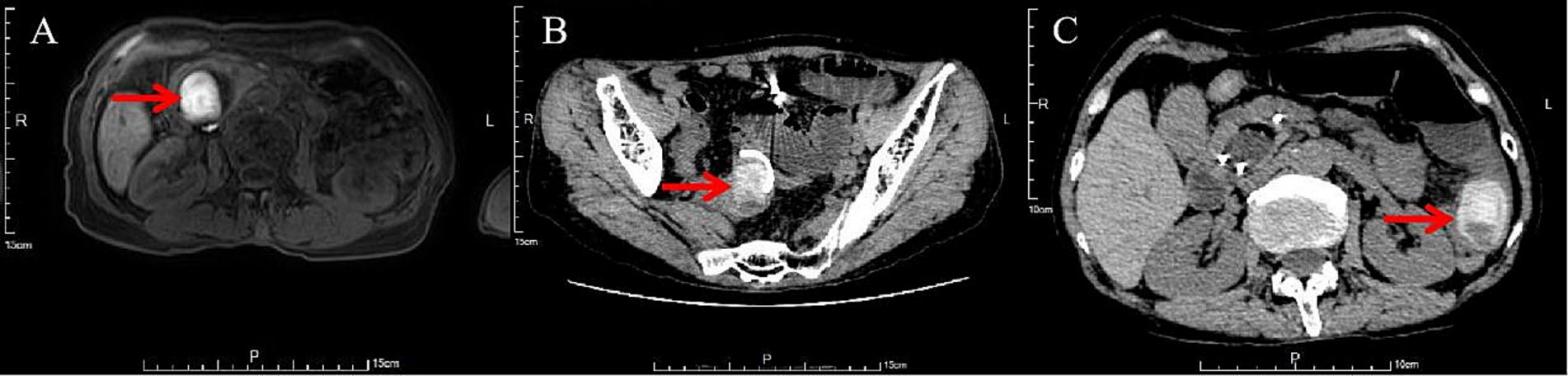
Changes in gallstone location (A) October 1 (MRI); (B) October 8 (CT); (C) October 14 (CT).
